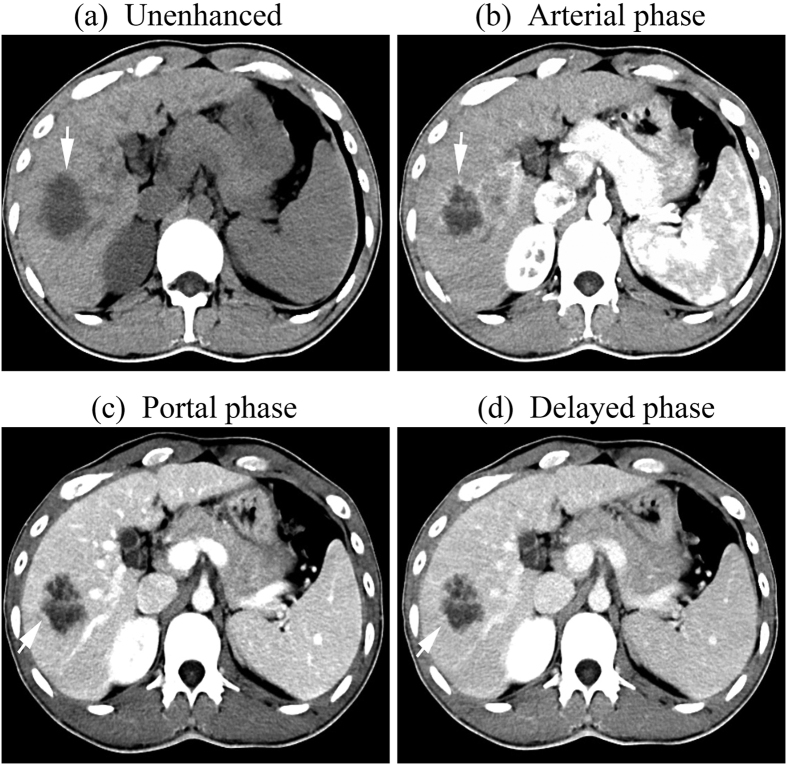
tif CT images of a 32-year-old man with a 4.2-cm –diameter ICC
